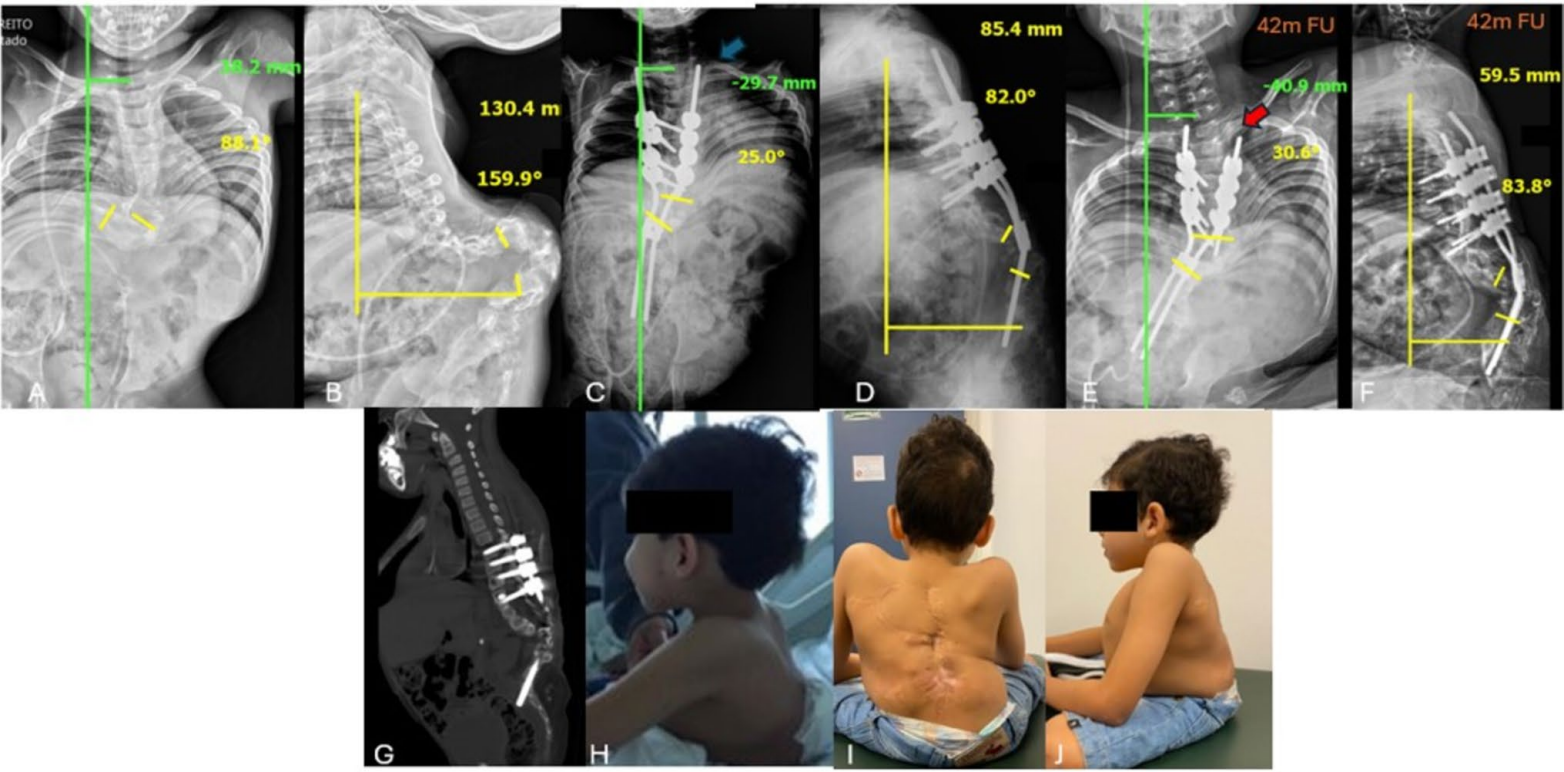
A, B AP and lateral preoperative radiographies showing serious lumbar kyphosis; C, D Immediate postoperative radiographies presents the length of the rod above the proximal screws [blue arrow], allowing trunk growth; E, F Panoramic radiograph on follow-up showing a decrease in the length of the rod above the proximal screws [red arrow), what was allowed by the self-sliding technique after 42 months of follow up; G Postoperative Sagittal CT; H Clinical feature of patient 2 before procedure; I, J Clinical feature of patient 2 at 42 months of follow-up
